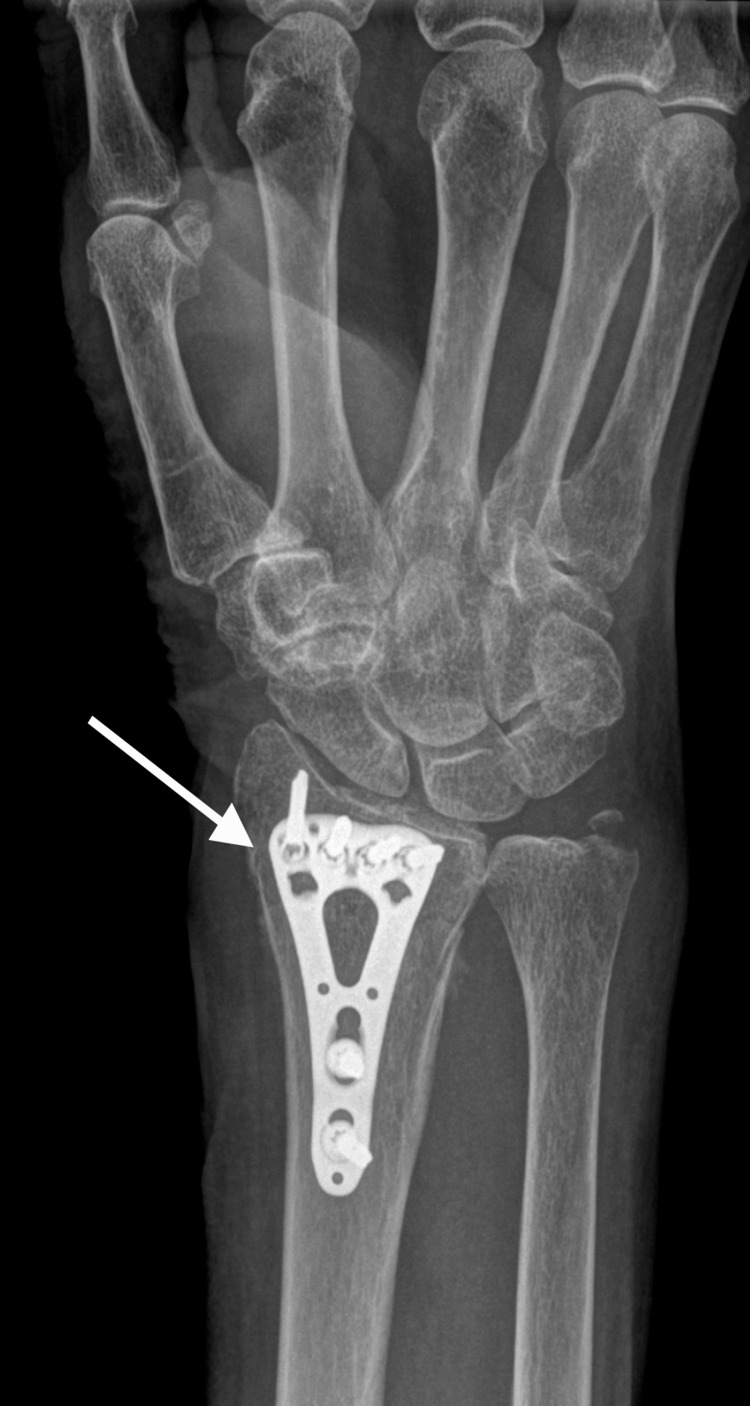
X-ray of the initial fracture at 14-month follow-up, anterior-posterior view, showing ongoing healing with encouraging signs of bridging callus formation
